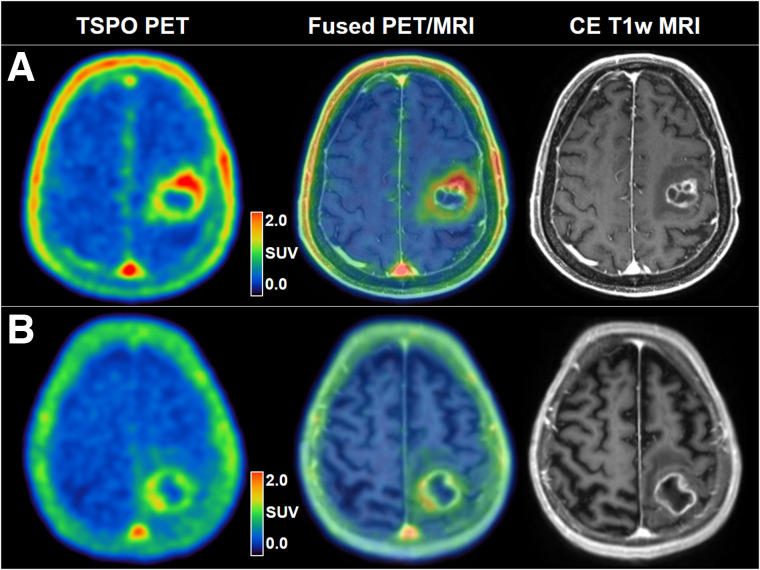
(A) 76-y-old male patient with left precentral IDH–wild-type glioblastoma (*MGMT* promoter-methylated, telomerase reverse transcriptase promoter C228T mutation; TSPO HAB) before hypofractionated radiotherapy and temozolomide chemotherapy. Tumoral TSPO radioligand uptake was high (SUV_max_, 2.4), and survival was short (OS, 4.5 mo). (B) 71-y-old female patient with left postcentral–parietal IDH–wild-type glioblastoma (*MGMT* promoter-methylated, telomerase reverse transcriptase promoter C250T mutation; TSPO MAB) before hypofractionated radiotherapy and temozolomide chemotherapy. Tumoral TSPO radioligand uptake was low (SUV_max_, 1.8), and survival was long (OS, 25.8 mo). CE-T1w = contrast-enhanced T1-weighted.
